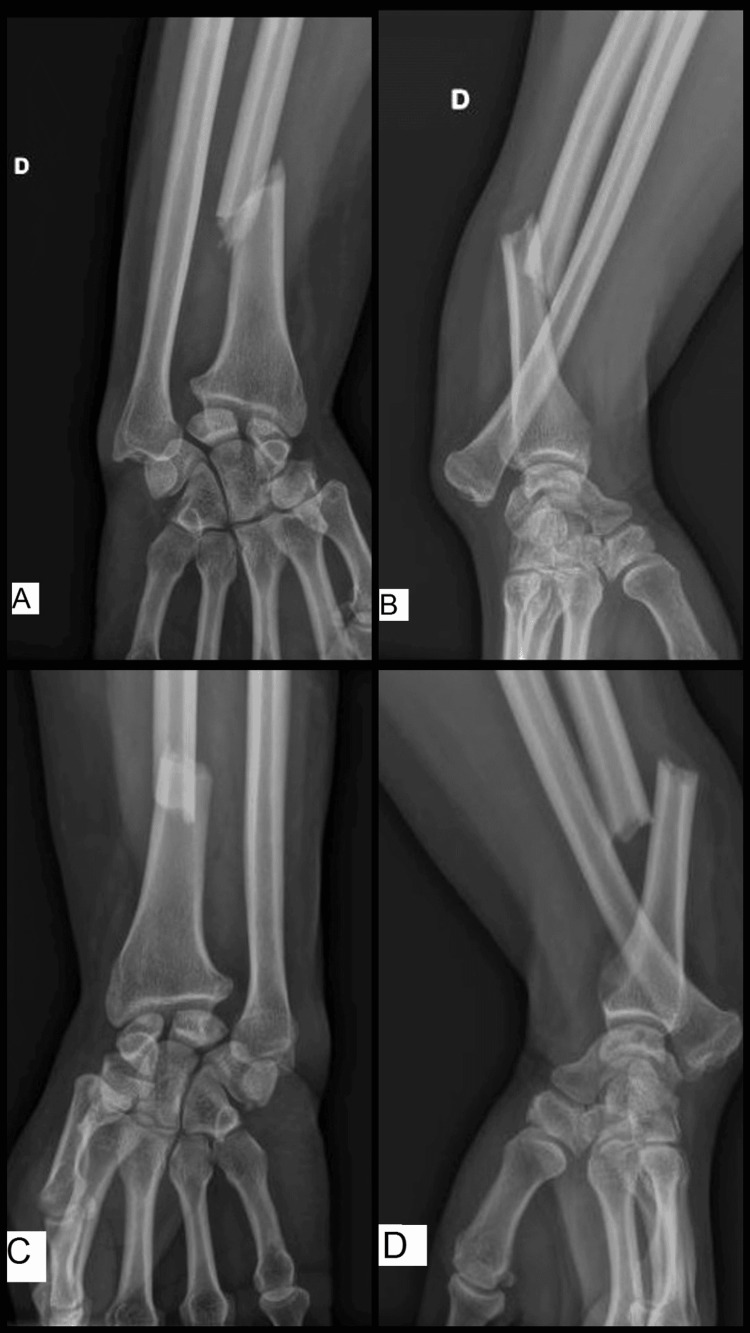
Preoperative X-rays Emergency room X-rays of the right wrist, including AP (A) and lateral (B) views, and X-rays of the left wrist, including AP (C) and lateral (D) views, demonstrating bilateral pronation type Galeazzi fracture dislocations.
